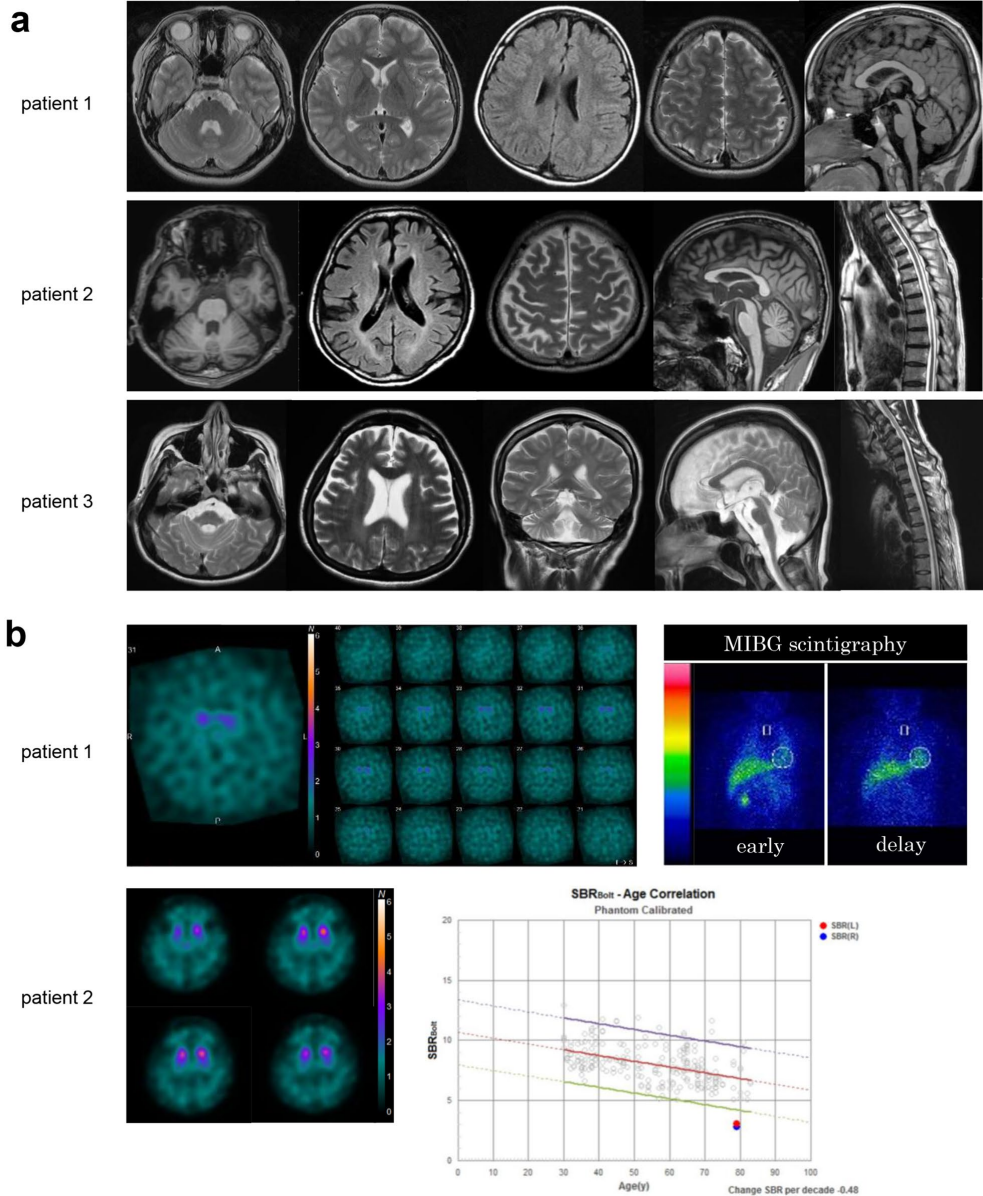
MRI findings, dopamine transporter SPECT, and MIBG scintigraphy. a Brain MRI of Patient 1 at the age of 11 years shows no obvious atrophy or significant white matter abnormalities in the cerebrum, brainstem, or cerebellum on T1-weighted, T2-weighted, and fluid-attenuated inversion recovery (FLAIR) images. Axial MRI images from Patient 2 show atrophy of the cerebellum, brainstem, spinal cord, and cerebral cortex, with a slightly high-intensity lesions in the bilateral cerebral white matter on FLAIR image. MRI of Patient 3 shows obvious atrophy of cerebrum, brainstem, and spinal cord, with mild bilateral hyperintensity in the periventricular white matter on T2-weighted image. b DAT scans of Patient 1 at the age of 11 years indicate bilateral decreased dopamine transporter uptake. MIBG scintigraphy shows normal uptake. DAT scans of Patient 2 at the age of 79 years show bilateral decreased dopamine transporter uptake
